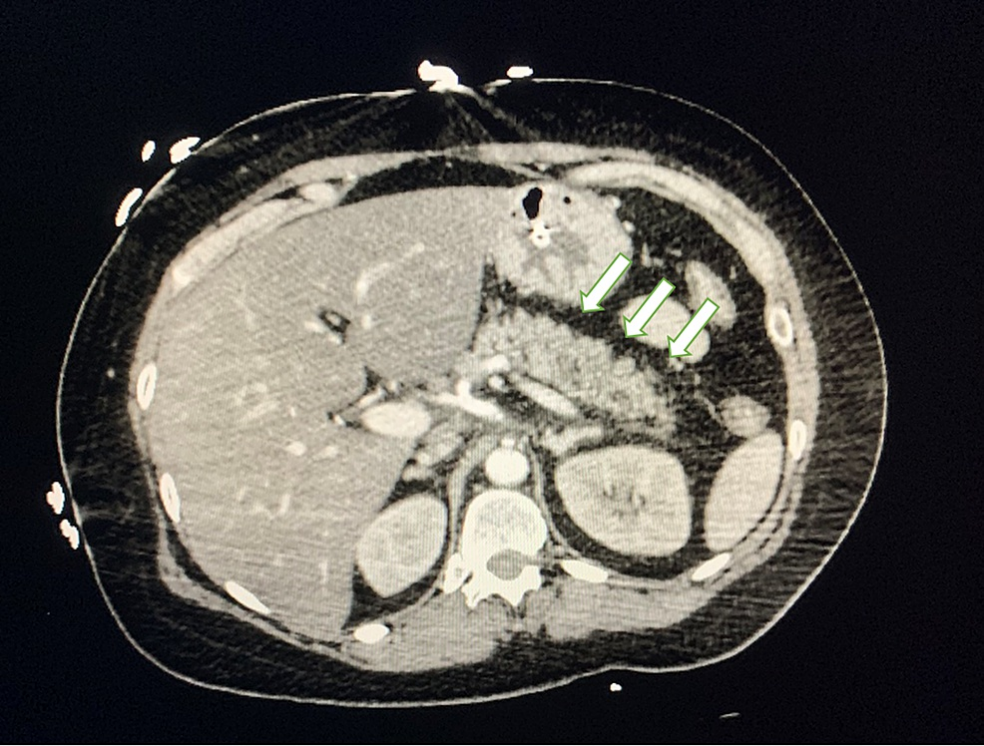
CT chest-abdomen-pelvis with IV contrast Acute pancreatitis with peripancreatic edema and fat stranding (white arrows). No peripancreatic collection or evidence of necrosis.
